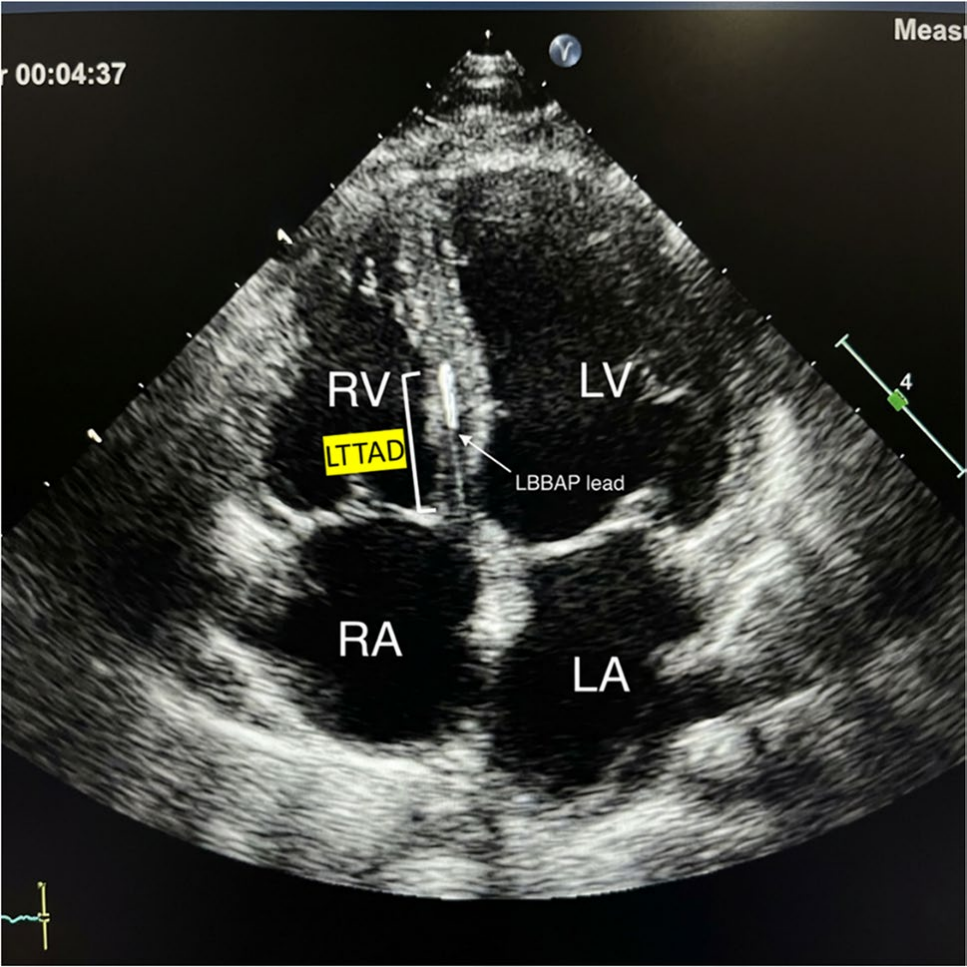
Measurement of echocardiographic distance between lead-implanted site and the tricuspid valve annulus (LTTAD)
